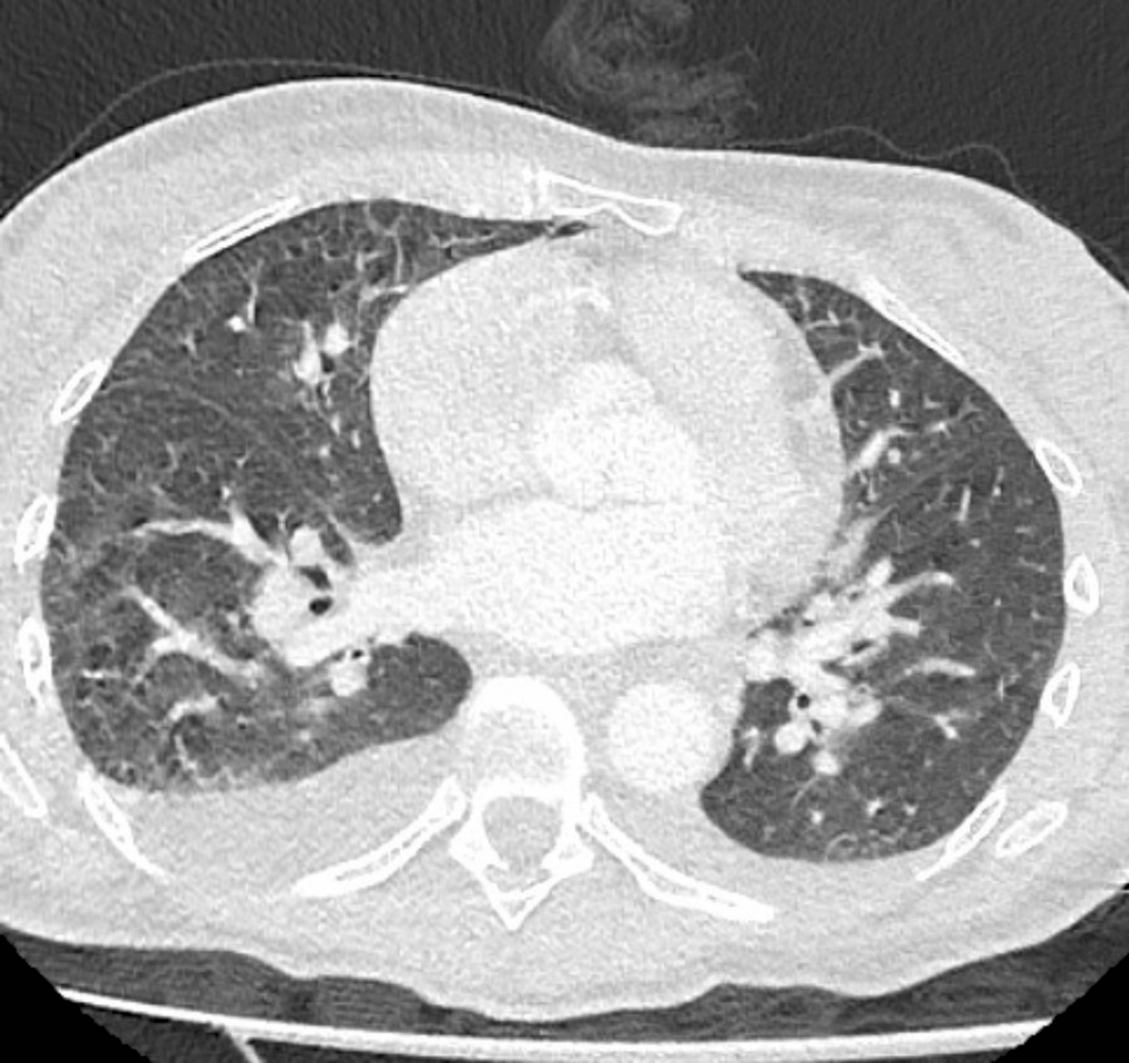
CT of the chest shows bilateral lower lung consolidation with small pleural effusions
